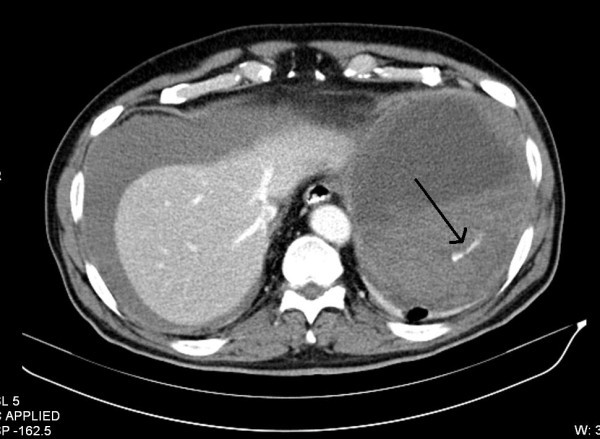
Abdominal computed tomography scans showing active bleeding in the splenic cystic lesion associated with signs of a recent massive hemorrhage and hemoperitoneum.
